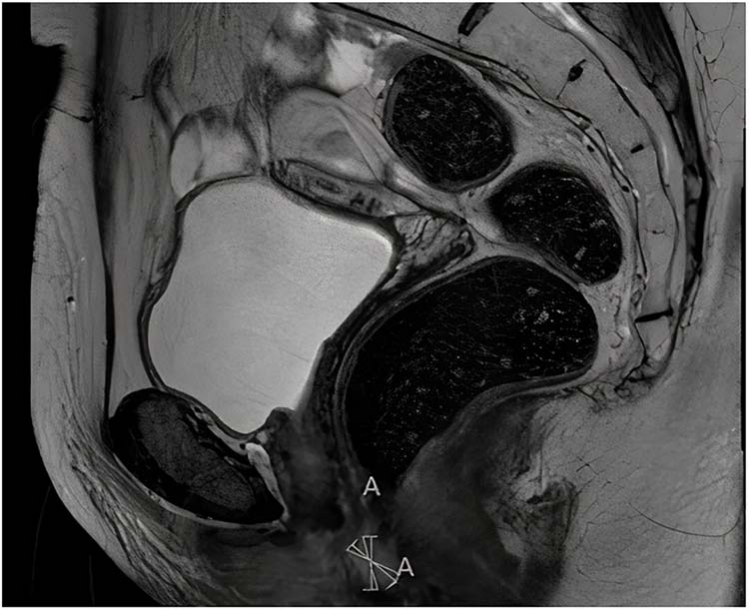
Sagittal view of diagnostic MRI-rectum with the solid tumour indicated with the linear mark ‘A’.
